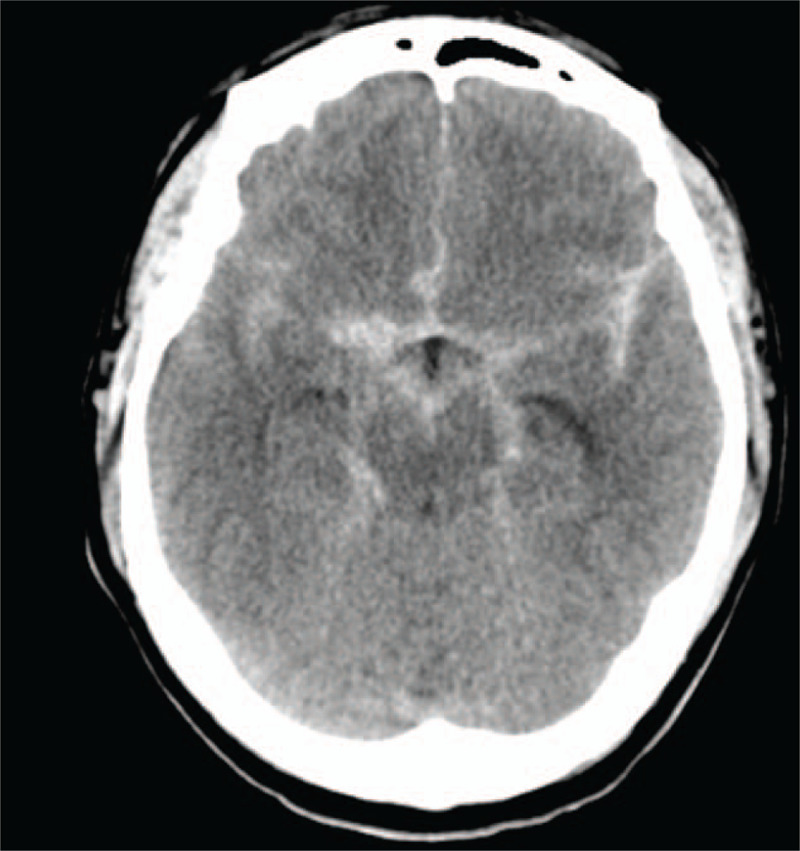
Brain computed tomography taken at the emergency room revealed SAH in the bilateral Sylvian fissure, basal cistern and interhemispheric fissure. SAH = subarachnoid hemorrhage.
